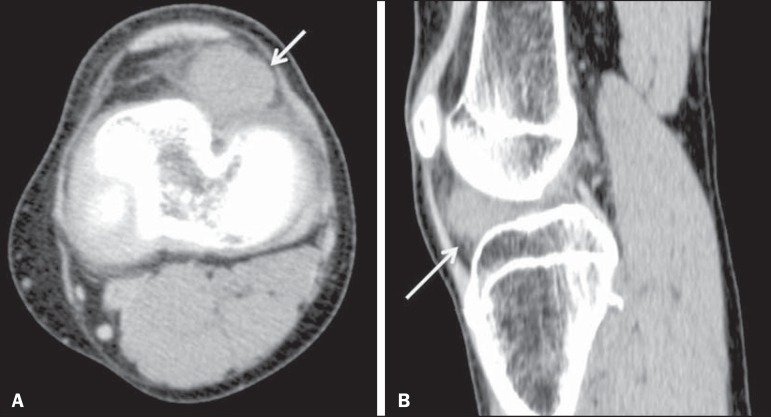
Intracapsular chondroma. CT scans acquired in the axial (A)
